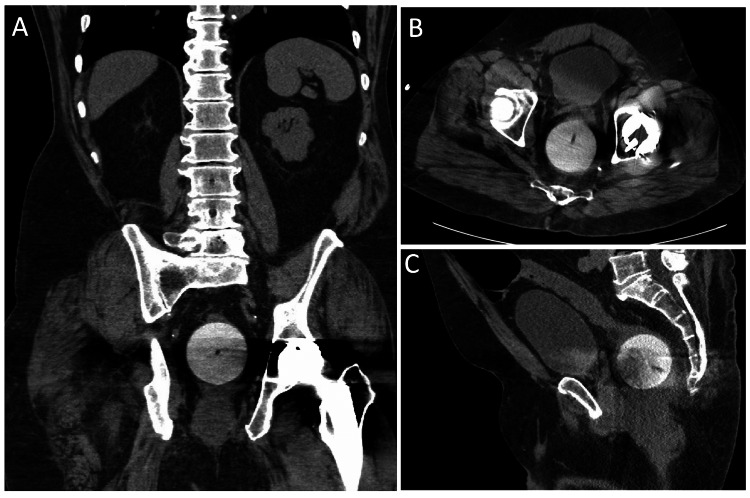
Representative computed tomography images of a silicon ball rectal foreign body seen in axial (A), sagittal (B), and coronal (C) projections.
